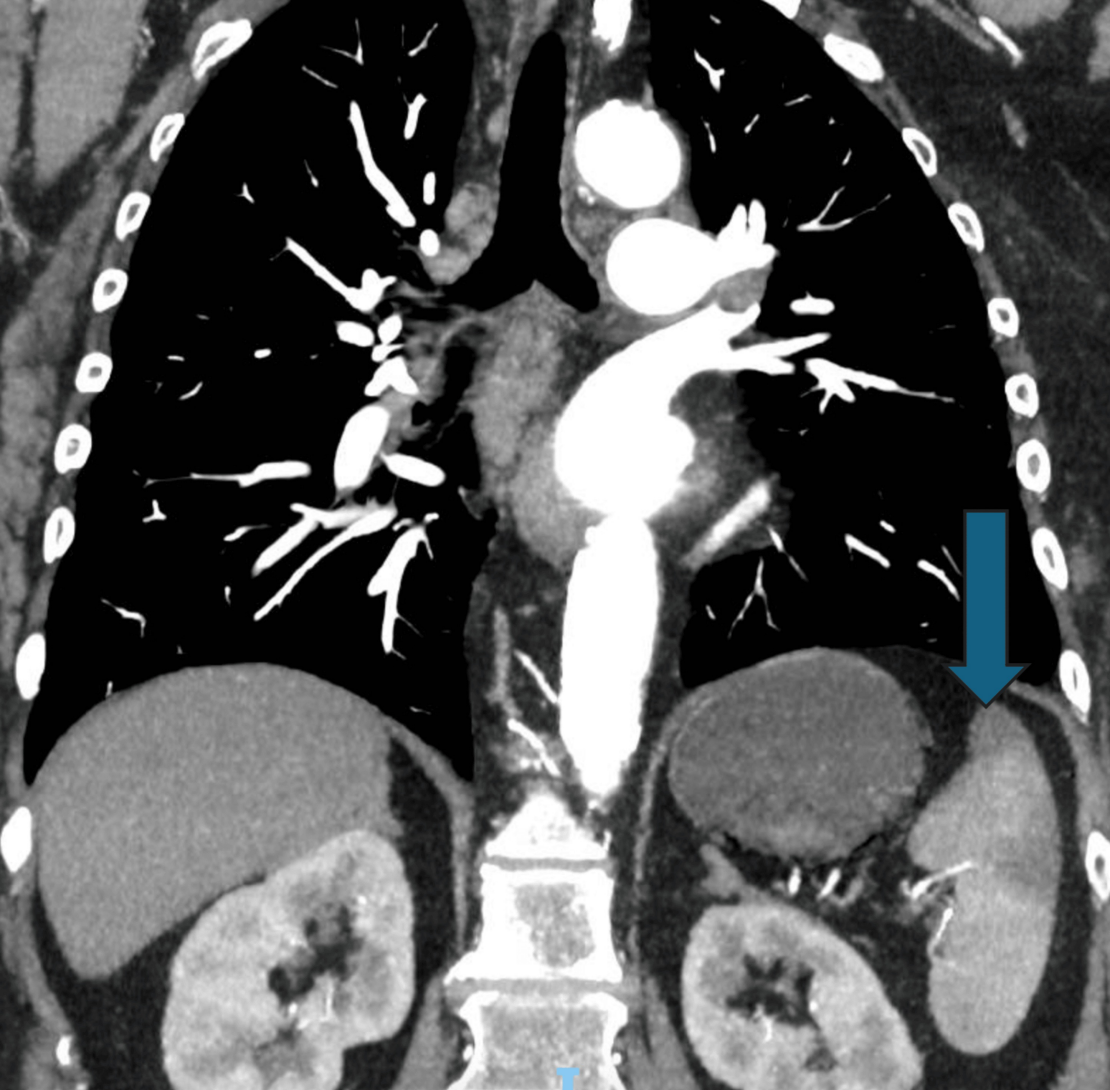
CT angiography showing the splenic infarct (arrow)
